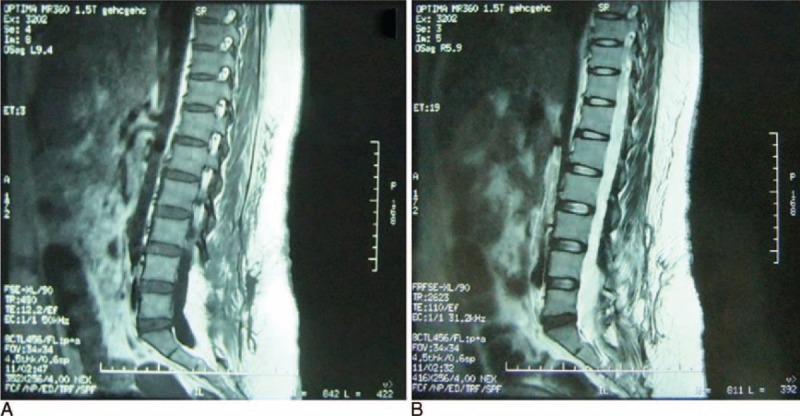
Postoperative MRI sagittal views of the lumbosacral region showing (A) ___ and (B) ___, with near disappearance of the watery area after 5 days of continuous suction drainage and 2 days of intermittent suction drainage. MRI = magnetic resonance imaging.
